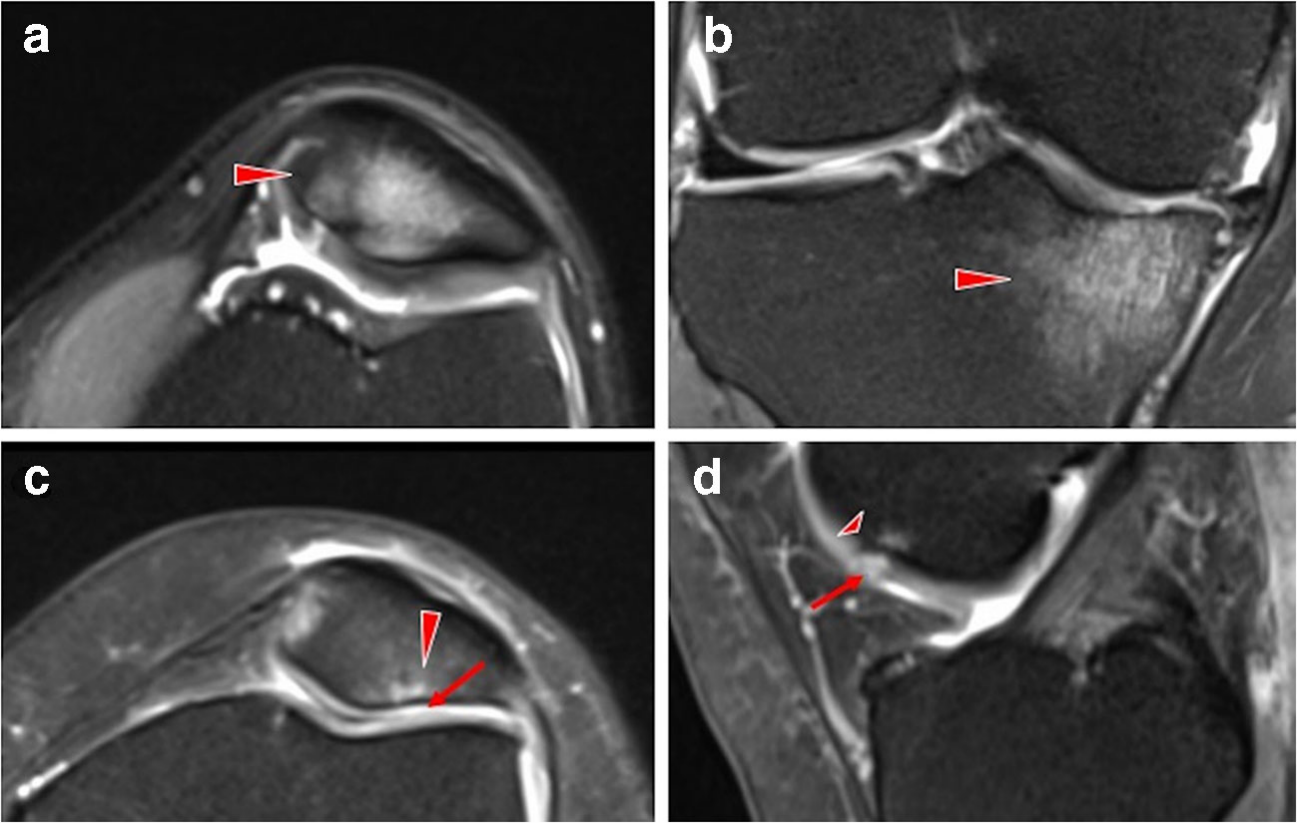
Axial proton-density fat-saturated MR images (a, c), coronal (b) and sagittal images (d) of high-grade bone marrow oedema lesion (grade 3: diameter ≥ 20 mm; in the (a) patella of the left knee of a 40-year-old man, (b) tibia of the right knee of a 59-year-old man; arrowheads) and high-grade cartilage defect (grade 4: full thickness defect exposing the bone; in the (c) patella of the left knee of a 44-year-old woman; arrow; with subchondral bone marrow oedema, arrowhead; (d) femur of the right knee of a 31-year-old woman; arrow; with subchondral ganglion cyst; small arrowhead)
